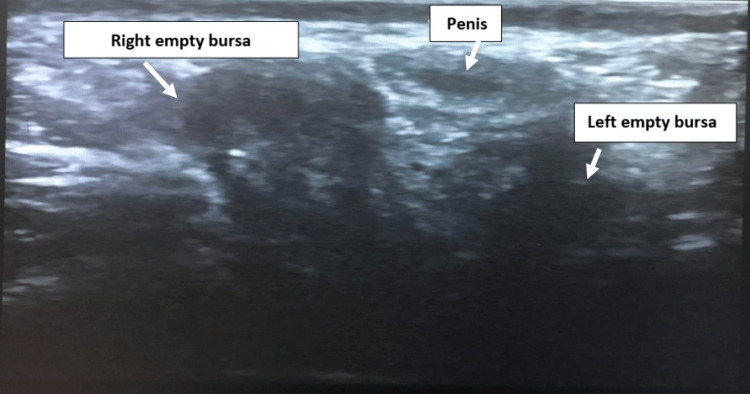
A transverse ultrasound image, in a ventral view through penis and bursae (arrows)
